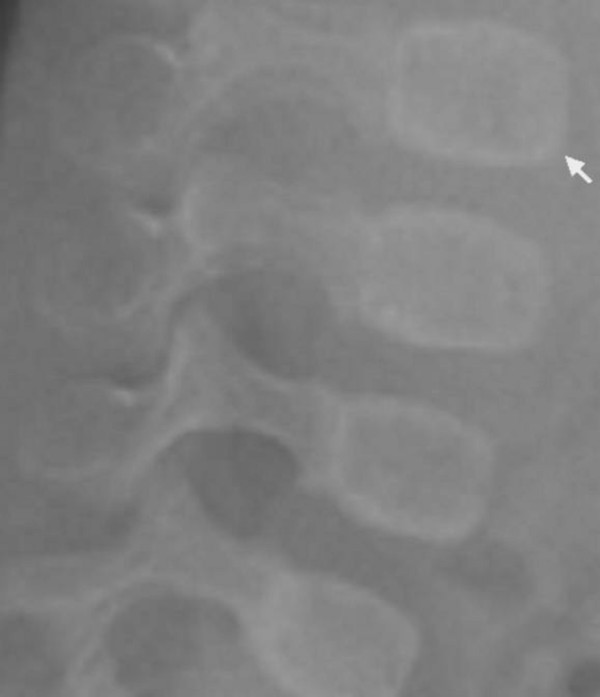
(patient 1). The lateral spine radiograph showed no platyspondyly, but marked sclerosis of the entire vertebral cortical rim (arrow) and unusual enlargement of the spinous processes.
